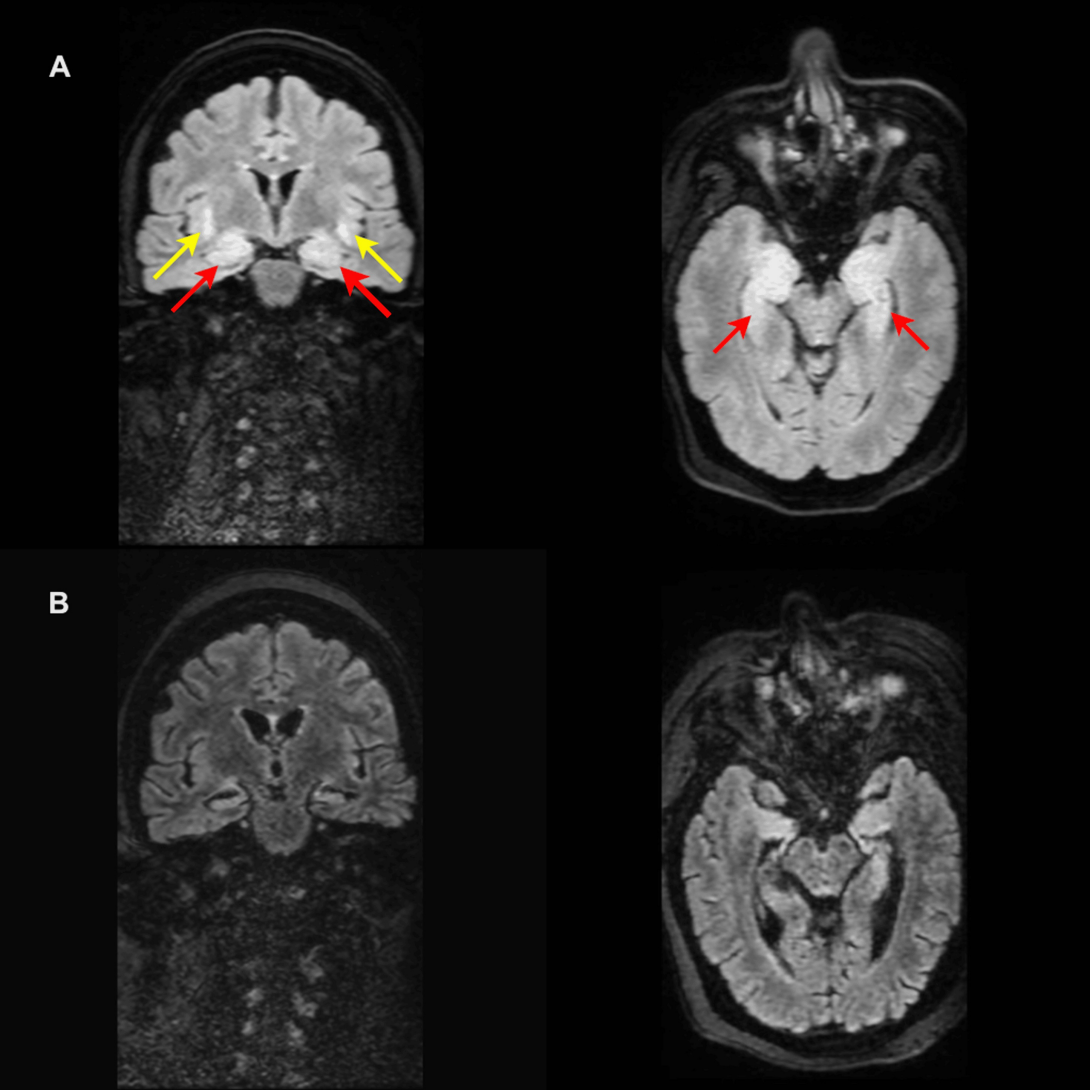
Serial brain MRI FLAIR A shows FLAIR hyperintensities affecting both mesial temporal lobes, including the hippocampi, and the amygdalae with enlargement bilaterally (red arrows), and involving subinsular regions (yellow arrows). B shows significant improvement in high signal intensity and reduction in swelling of FLAIR hyperintensities. FLAIR: fluid-attenuated inversion recovery.
